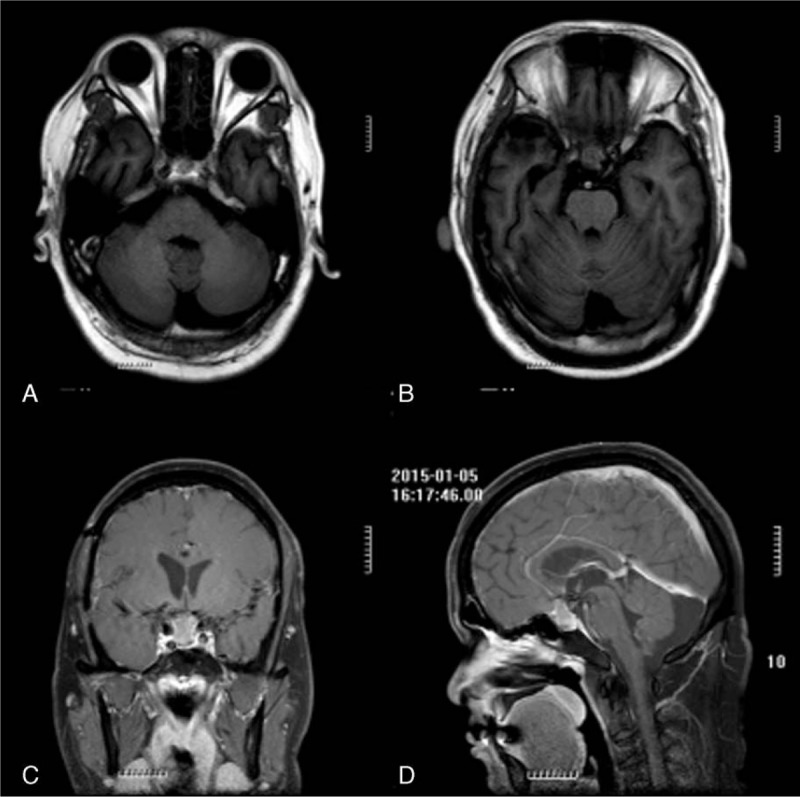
MRI suggested that the tumor size was 1.2 cm × 1.1 cm × 1.7 cm with the integrative therapy of traditional Chinese herbal decoction and brucea soft capsules (January 2015) (A–D).
